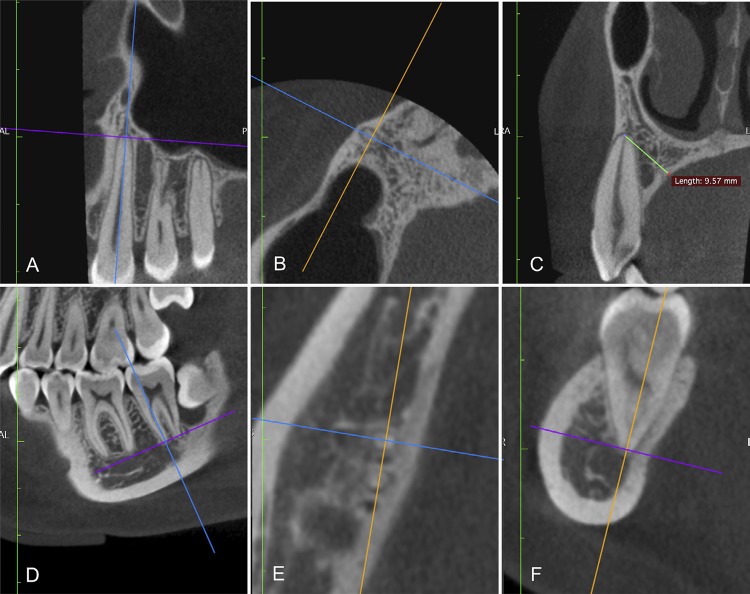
CBCT images in the sagittal, axial, and coronal planes (A-F). Standard reference for the location of the apical foramen was the main root canal. Axial navigation was used for each root individually
